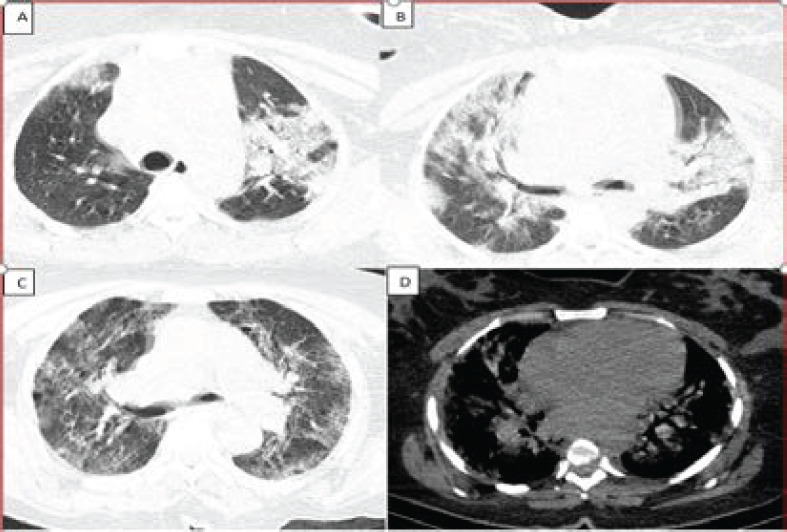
Axial lung (A, B, C) and mediastinal(D) chest HRCT windows of a 32-year-old female patient showing bilateral multilobar ground glass opacities and airspace disease with a peripheral predominance, as well as diffuse interlobular septal thickening. This patient was categorized as CORADS 5, stage 3 with a severity score of 17/25
